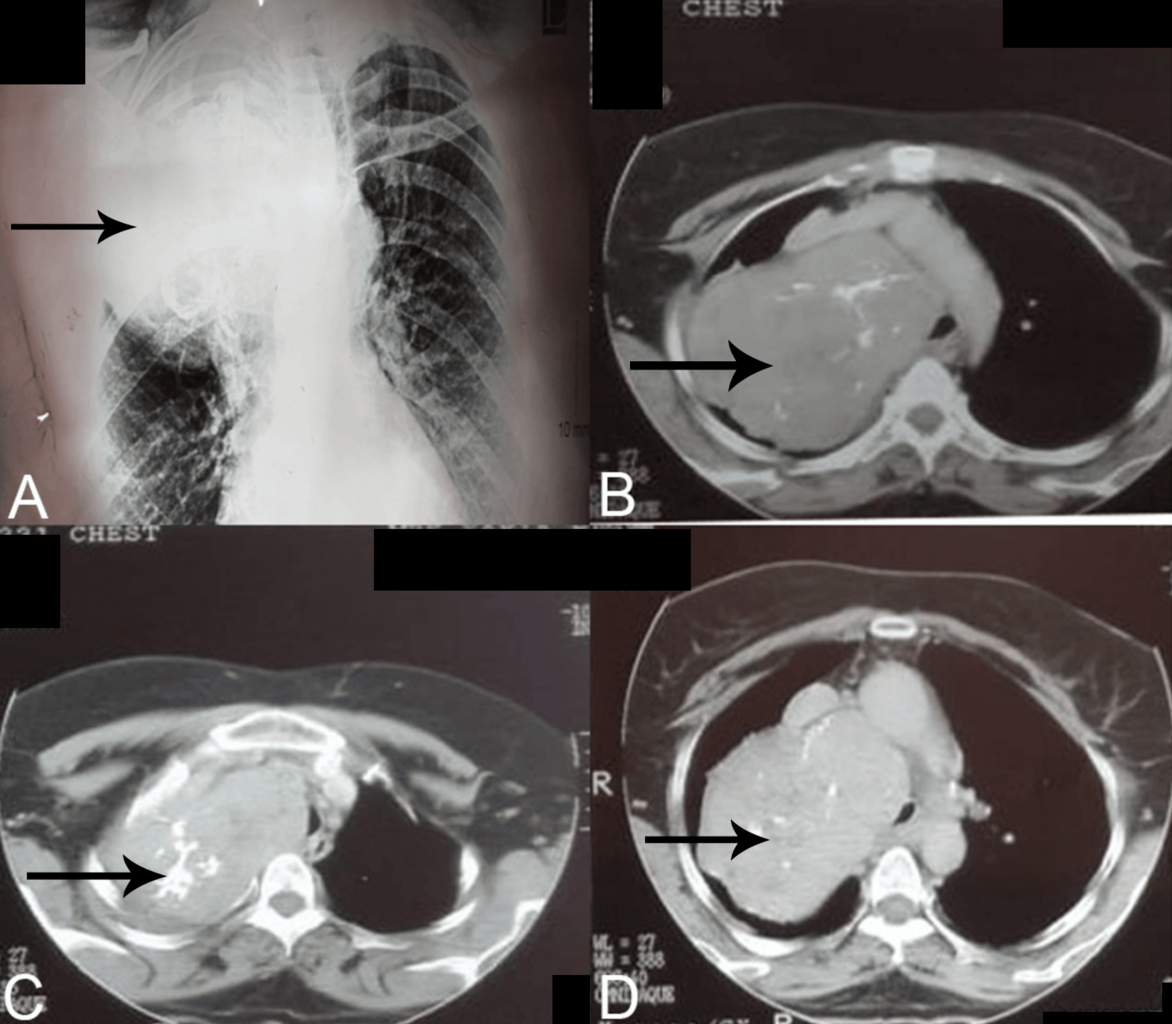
Radiological investigations of the patient. (A) X-ray showing right-sided mass in the region corresponding to the apex of the lung. (B-D) CT scan sections of the patient showing growth in the right side of the thorax.
